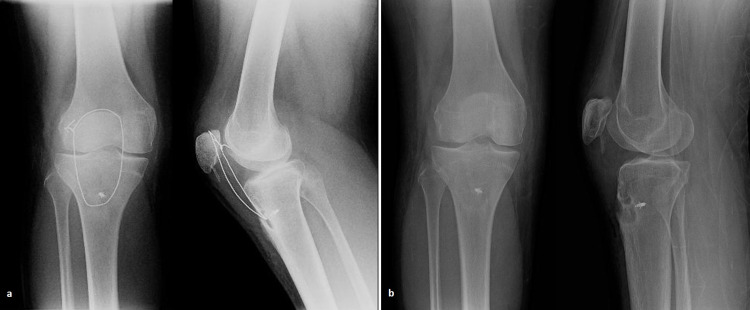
Postoperative and late follow-up radiographs. (a) Postoperative anteroposterior and lateral radiographs at one-week follow-up showing correction of patella alta; (b) final follow-up radiographs at one year showing maintenance of the reduction.
